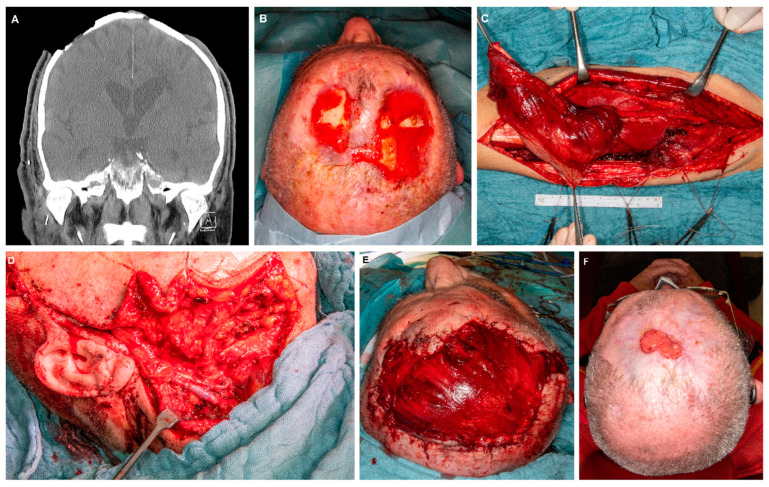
Example of a cranial reconstruction using a vastus lateralis muscle flap in a patient with two cutaneous squamous cell carcinomas of the parietal region. (A): CT scan presenting the primary tumor region with infiltration of the calvarial bone. (B): Image of the preoperative situation after partial tumor resection. (C): Intraoperative situation of the harvesting procedure of the muscle flap. (D): Intraoperative situation after anastomosis of the pedicle vessels to the superior thyroid arterial and the facial artery. (E): intraoperative situation after soft tissue reconstruction with the vastus lateralis muscle flap. (F): situation after 9 months during secondary healing (i.e., conservative therapy via wound coverings until epithelialization).
